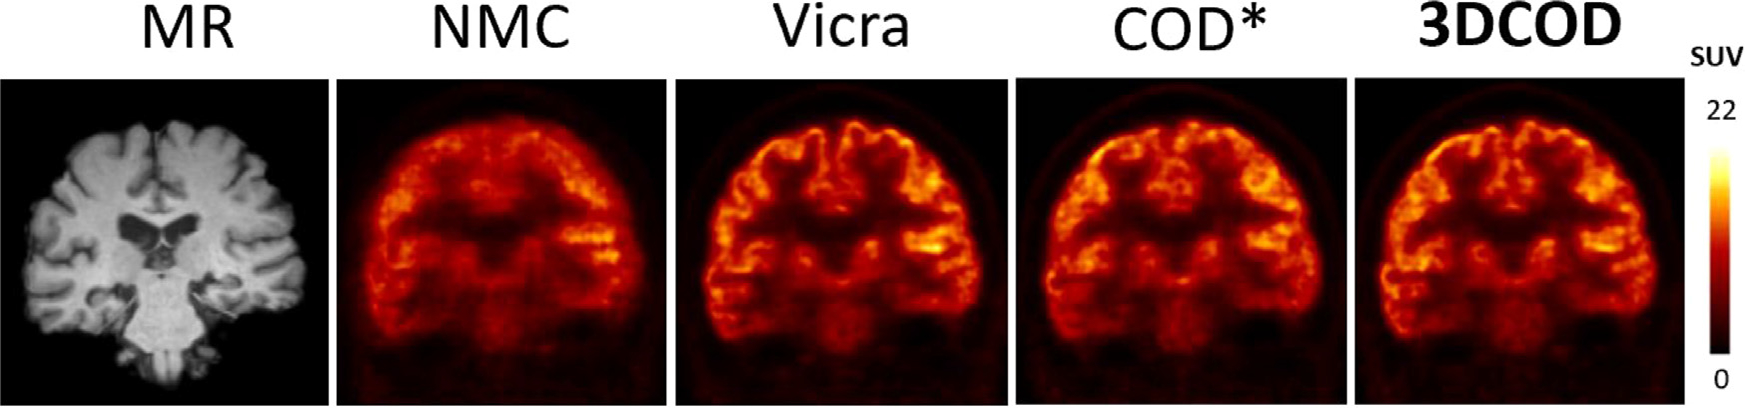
Sample slices of motion-corrected reconstructions of a real ^18^F-FDG (60–90 min) HRRT study showing the proposed 3DCOD approach compared with NMC, Vicra and COD* (example result from our previous work).
